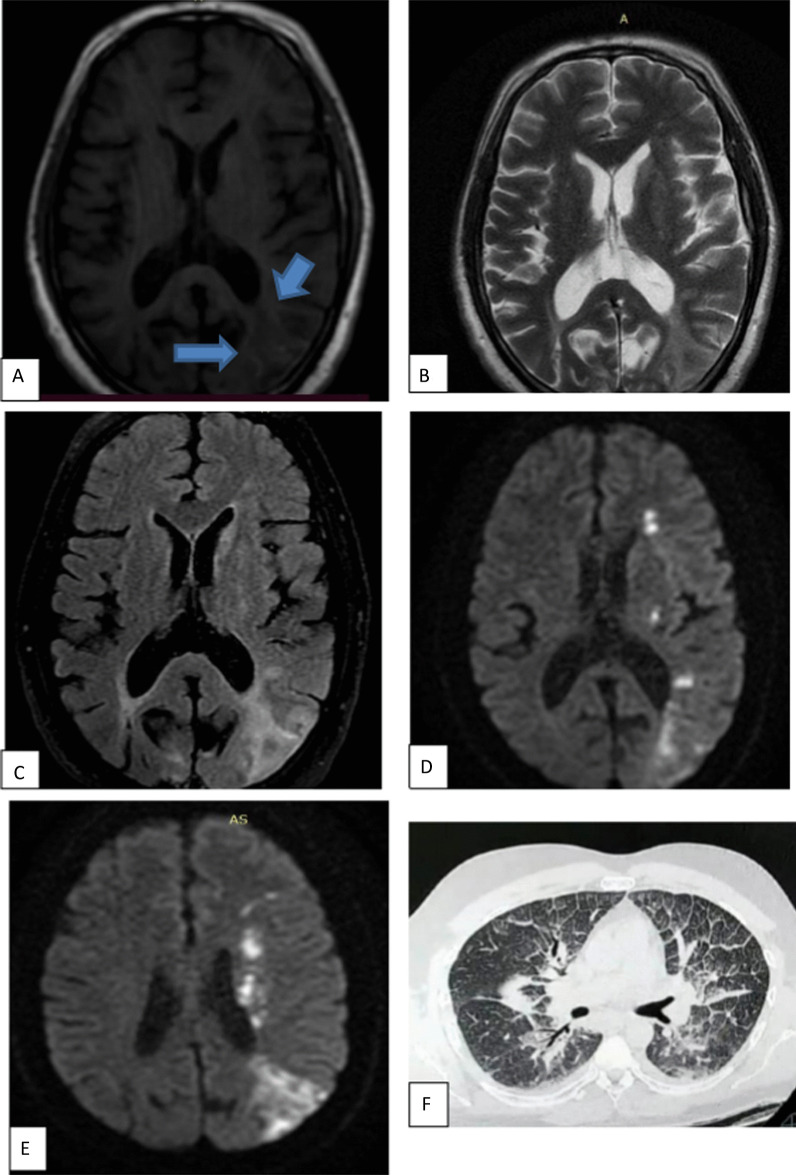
A 43-year-old female patient presented with post COVID fatigue, followed by cerebrovascular stroke symptoms. MRI axial T1WI (a) revealed left occipital subcortical white matter abnormal signal display relative low signal intensity (arrow). Axial T2WI (b) show high SI with no mass effect. Axial FLAIR (c) showed corresponding increase signal intensity. DWI (d, e) showed areas of restricted diffusion. watershed infarcts with additional foci of lacunar infarcts. CT lung showed GGO very typical for COVID (CORAD-V)
